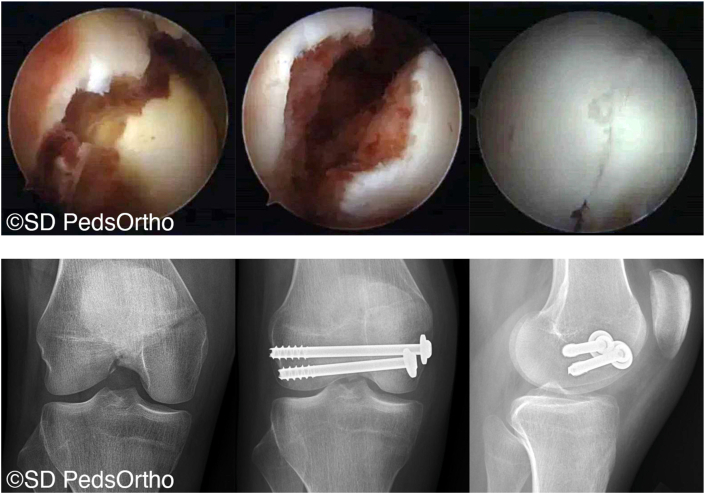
Prereduction (top row left and center image) and post-reduction (top row right image) arthroscopic images and pre- and postoperative radiographs (bottom row).
